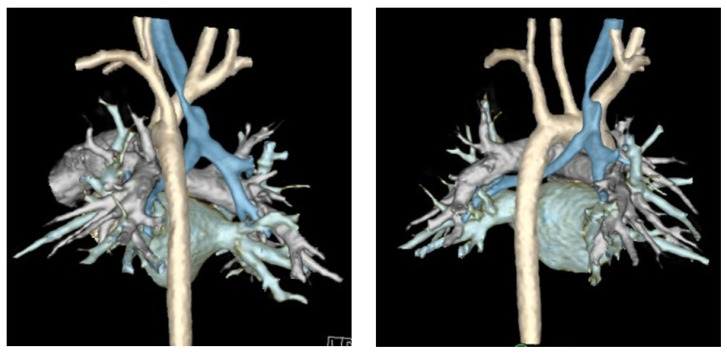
Three-dimensional reconstruction of dynamic airway CT scan. Trachea is highlighted in blue and aorta is highlighted in off-white/cream.
